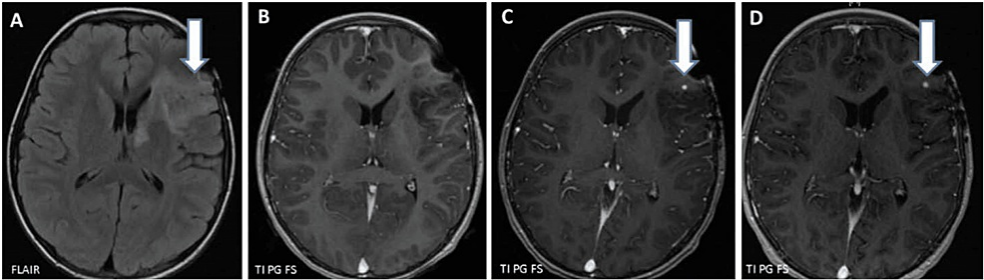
Axial MRI images. Initial axial MRI (A) shows a hyperintense left frontal lobe oligodendroglioma that was resected at age six years. No post-contrast enhancement was seen in the resection bed on the T1-weighted post-gadolinium axial image two years after the surgery. T1-axial post-contrast axial MRI image (C) performed six months after image (B) shows a tiny 2-mm enhancing focus (arrow) in the surgical bed that remained stable even after two more years (D). MRI: magnetic resonance imaging.
